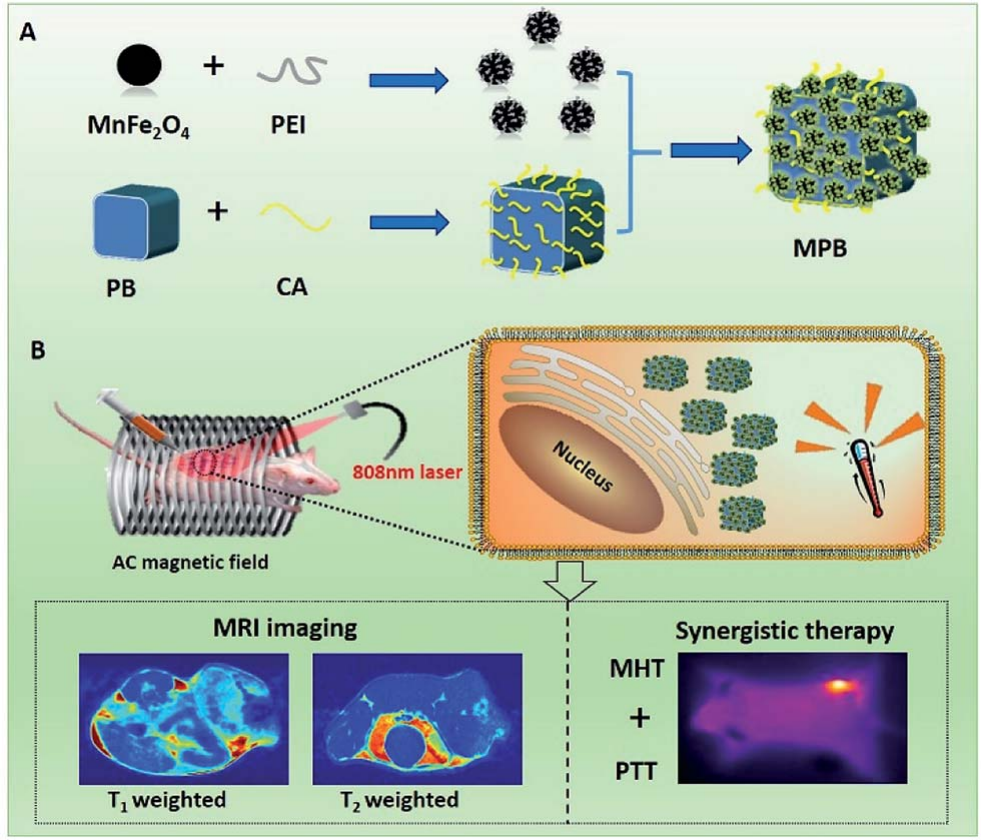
Synthesis of MPB NPs and their applications for *T*_1_/*T*_2_ MRI-guided synergistic photothermal/magnetic hyperthermia.
